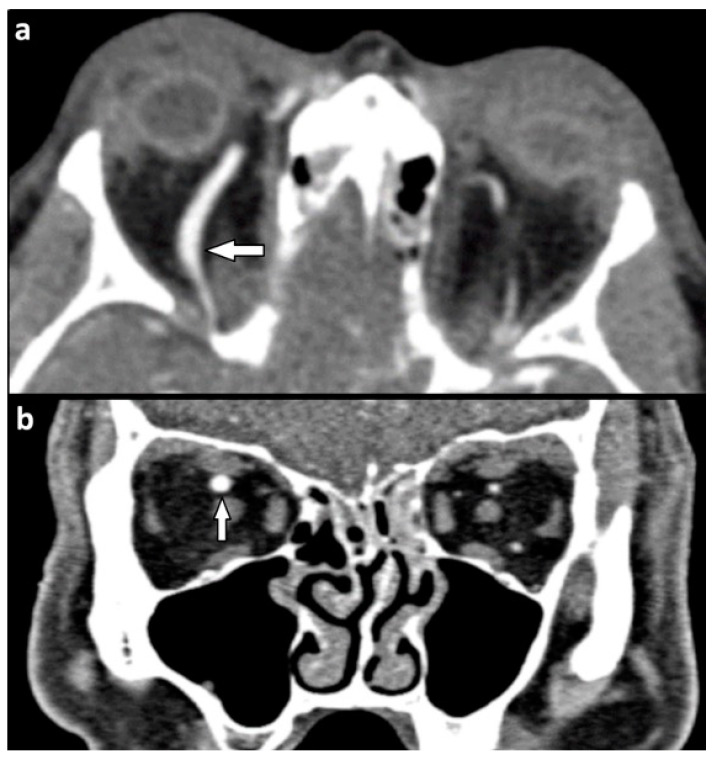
Prominent right ophthalmic vein (white arrows). Compared to the contralateral vein, the vessel appears engorged. CE-MSCT, soft tissue window. (a) Axial reformation. (b) Engorged superior ophthalmic vein and exophthalmos of the right globe hinting at post-traumatic CCF (CE-MSCT = contrast-enhanced multi-slice CT, CCF = carotis-cavernous fistula).
